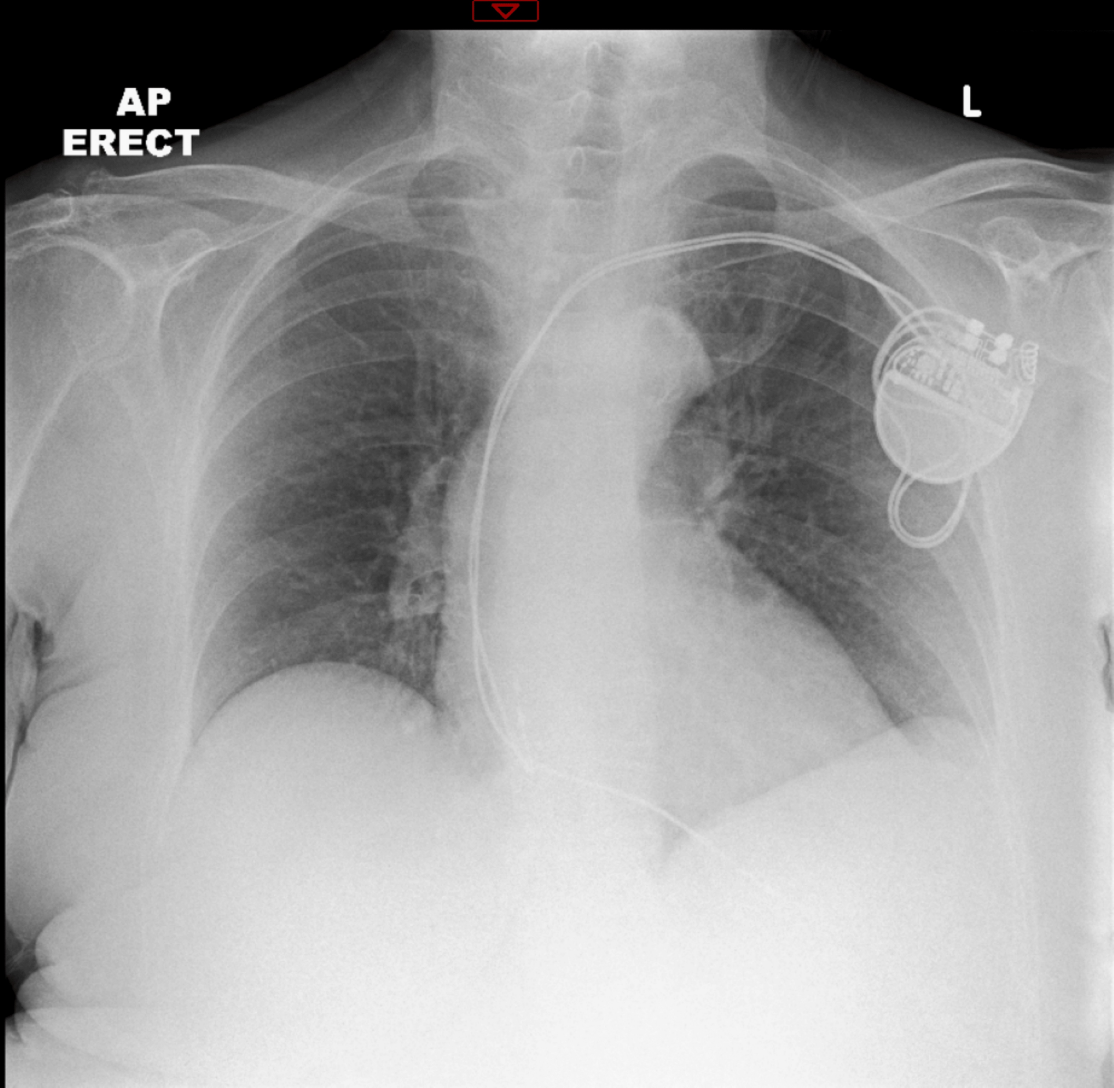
Patient's chest X-ray (anterior to posterior) demonstrating no acute abnormalities with a dual-chamber cardiac pacemaker in situ
